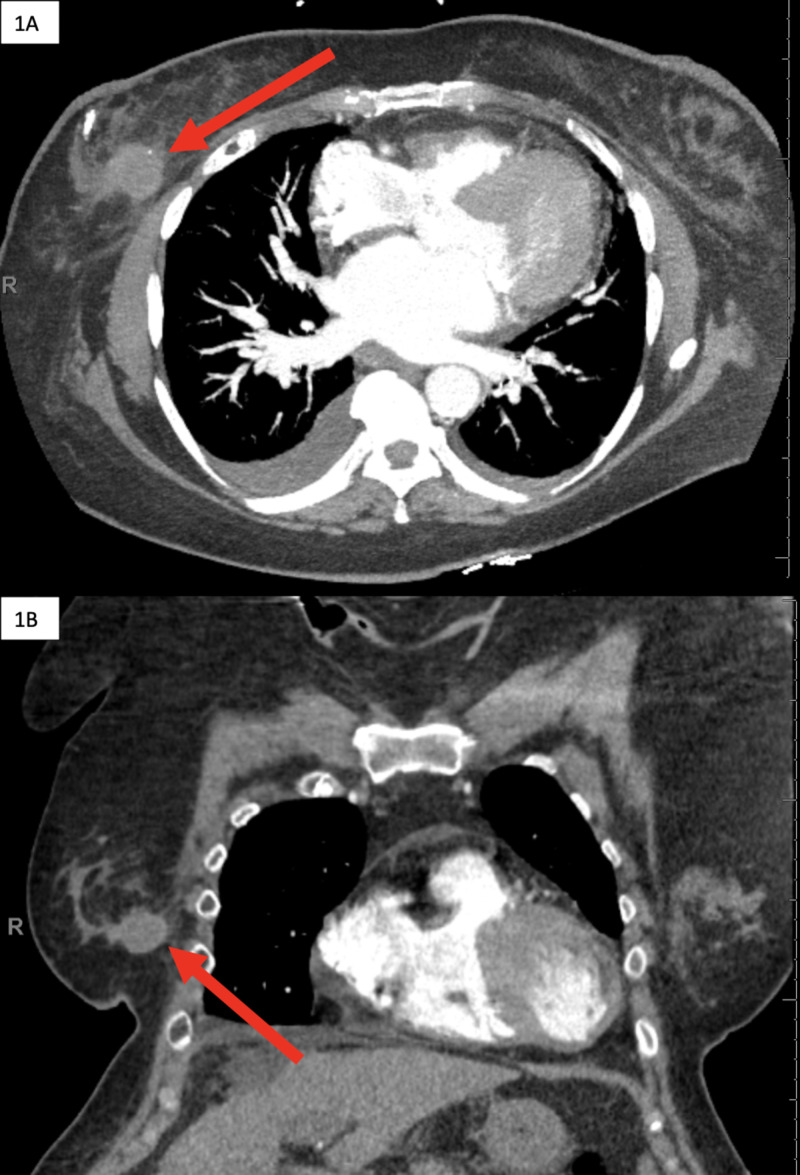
Pulmonary angiogram chest CT performed in the emergency department Axial (A) and coronal (B) images demonstrate incidental finding of isoattenuating mass with subtle rim enhancement (red arrows) in the right breast.
